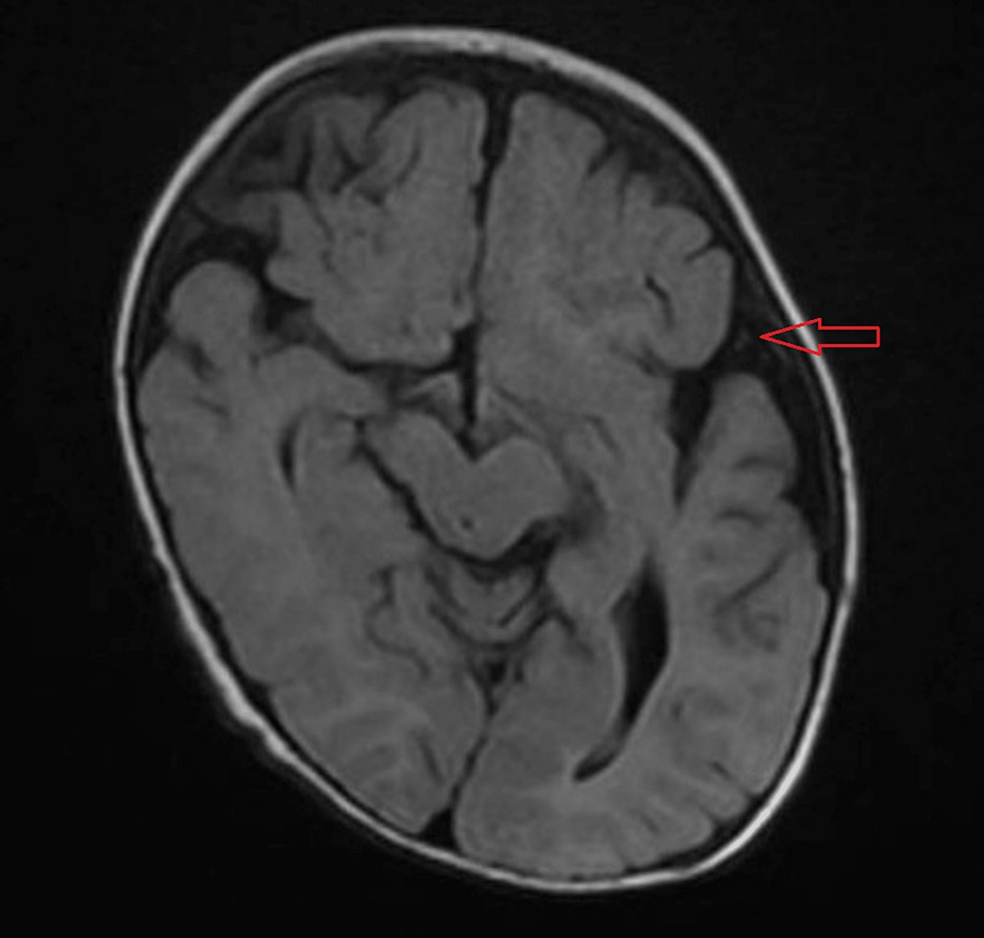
MRI brain without contrast Left hemicerebrum atrophy, as evidenced by the prominent ventricular system and extra-axial CSF spaces on the left side, most likely secondary to a brain insult (arrow)
